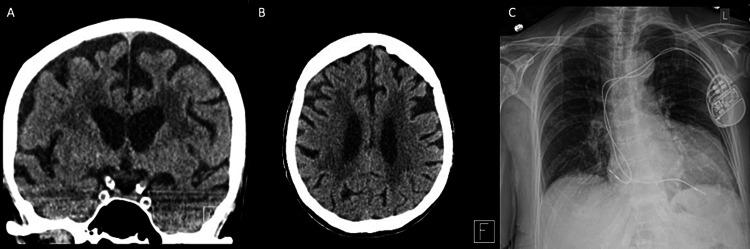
Radiographic imaging CT of the head without contrast in (A) coronal and (B) axial views. (C) AP chest X-ray. Imaging findings were unremarkable for any acute changes that would explain the patient’s hallucinations AP: anteroposterior; CT: computed tomography
